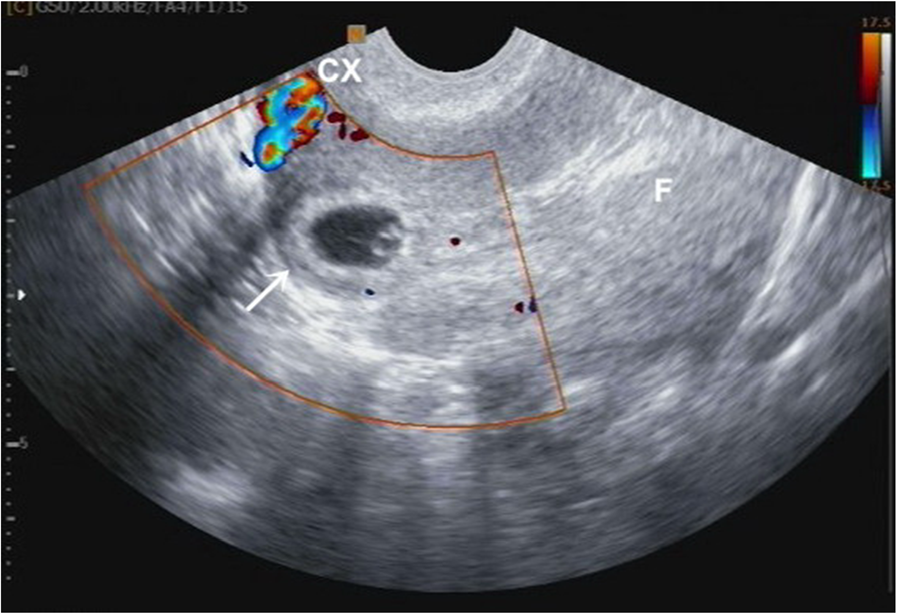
A cesarean scar pregnancy demonstrated by ultrasound showing the gestational sac implanted in the lower segment dehiscence of the anterior myometrium. The residual myometrium was thin and the amniotic sac bulged into the uterovesical fold under the cesarean scar (indicated by the arrow). CX: cervix; F: fundal endometrial cavity
